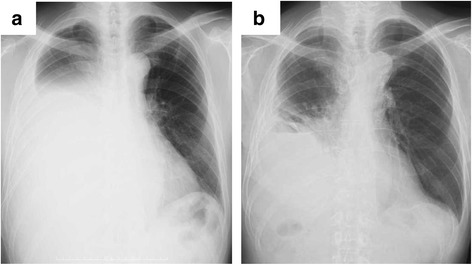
a Chest roentgenogram at presentation showing right pleural effusion. b Chest roentgenogram 2 months after right pleurectomy/decortication (P/D) following *left upper* division segmentectomy
